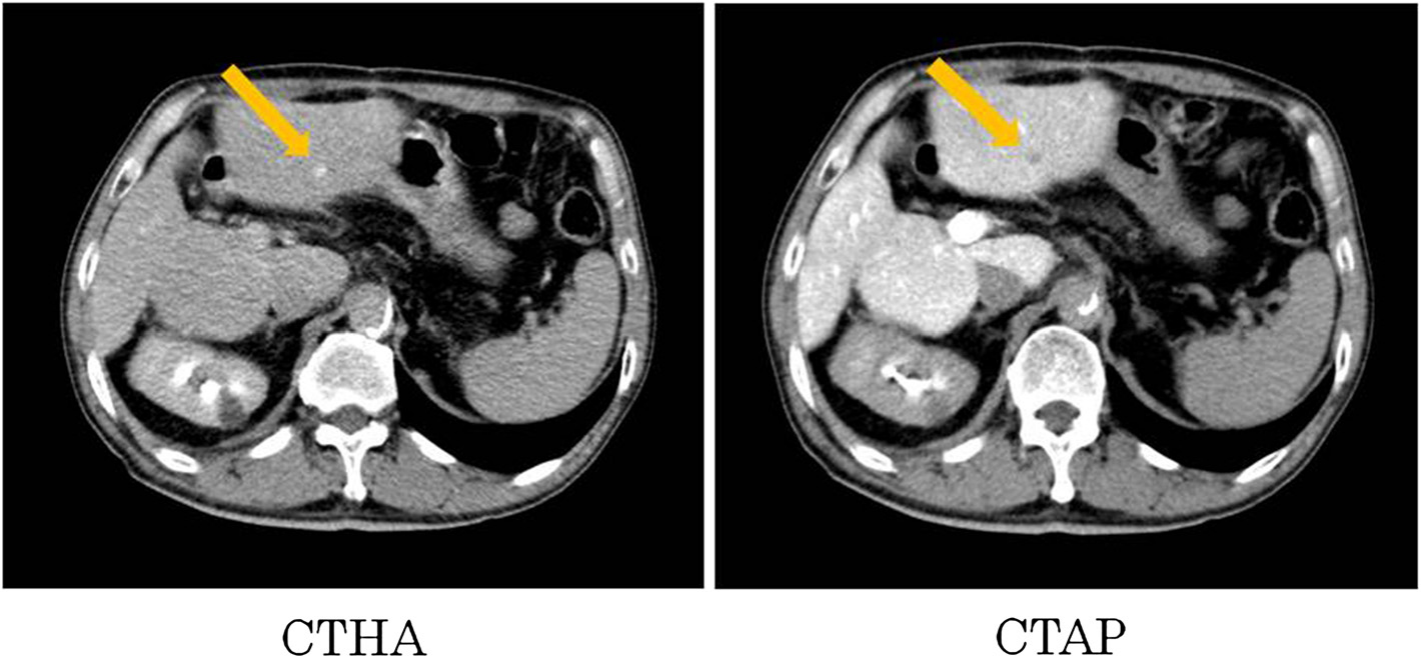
Computed tomography angiography revealed a 9-mm-diameter intrahepatic recurrence in segment 3.
